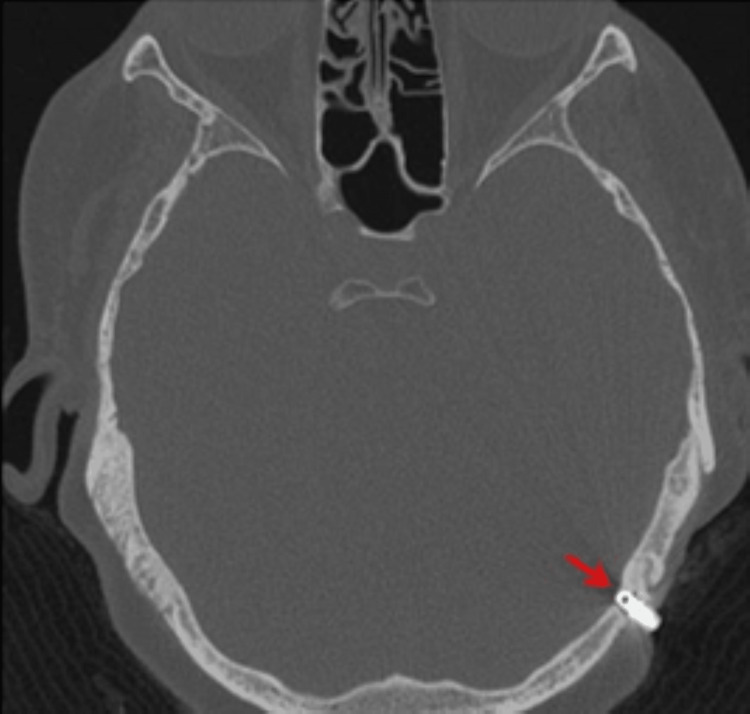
CT scan showing bone loss at the left implant site one month prior to extrusion on November 23, 2013
